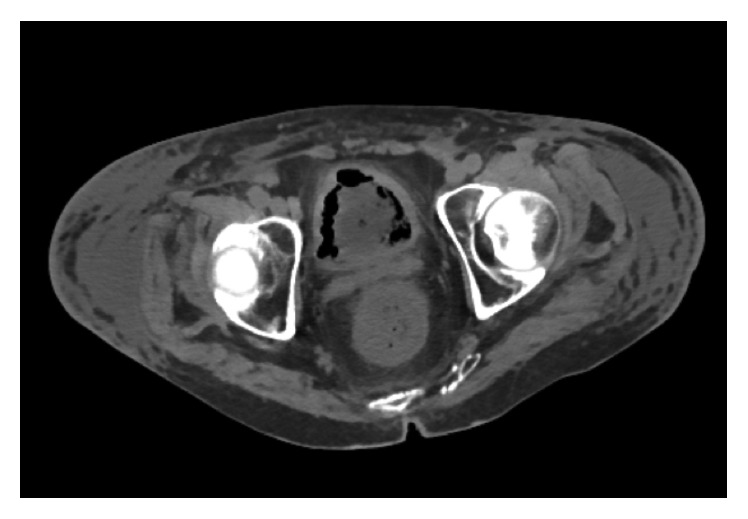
CT abdomen (transverse view) demonstrates nonspecific density and foci of air noted in bladder.
